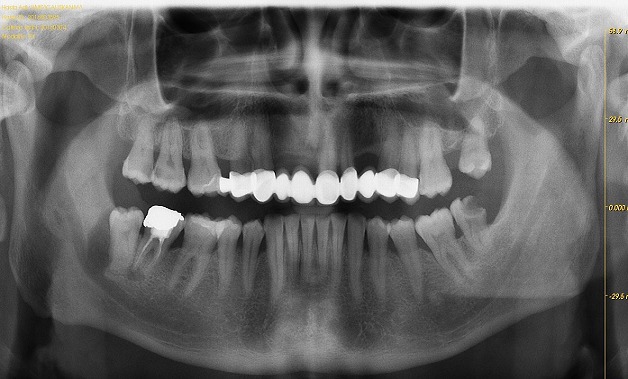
Preoperative panoramic radiograph of the lesion
